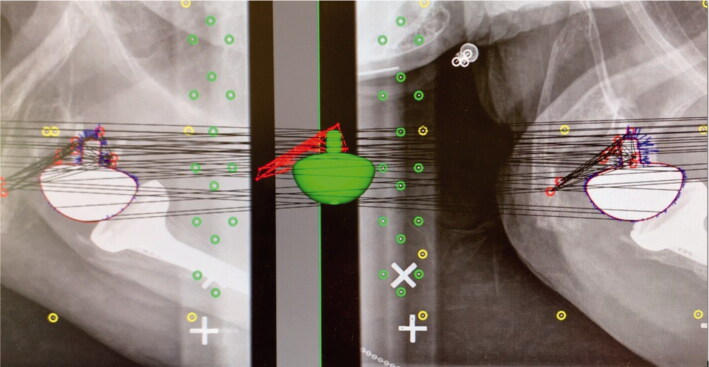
Radiostereometric analyses were performed with RSAcore software using reversed engineering (RE). The virtual glenoid implant (green) corresponds with the red demarcated outline of the actual glenoid implant shown on dual simultaneous radiographs of a right-sided shoulder with a reverse total shoulder arthroplasty. RSA markers are red, control markers are green, and fiducial markers are yellow.
